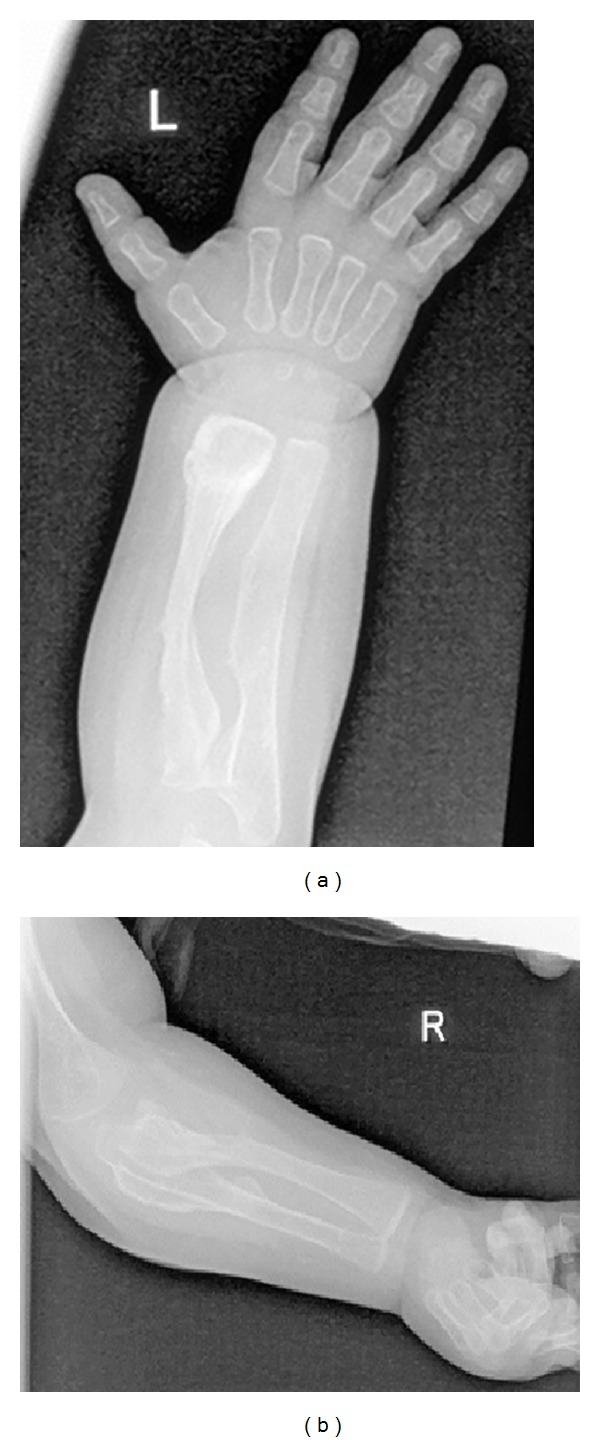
Anteroposterior upper limbs radiograph showed bilateral calcified interosseous membranes of the forearms associated with bilateral anterior subluxation of the radial heads; the ends of the long bones were thick. Osteoporosis and broad and short bones of the hands and the carpal bones were small and dysplastic associated with apparent swelling of the forearms.
